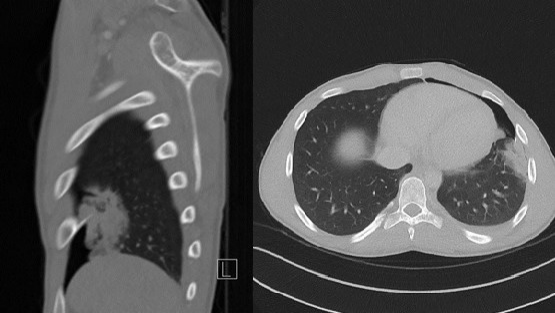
Sagittal and axial reconstruction CT angiography (in bone and pulmonary setting respectively), showing an osseous proliferation perforating the lung parenchyma causing a pneumothorax and pulmonary hemorrhage.
